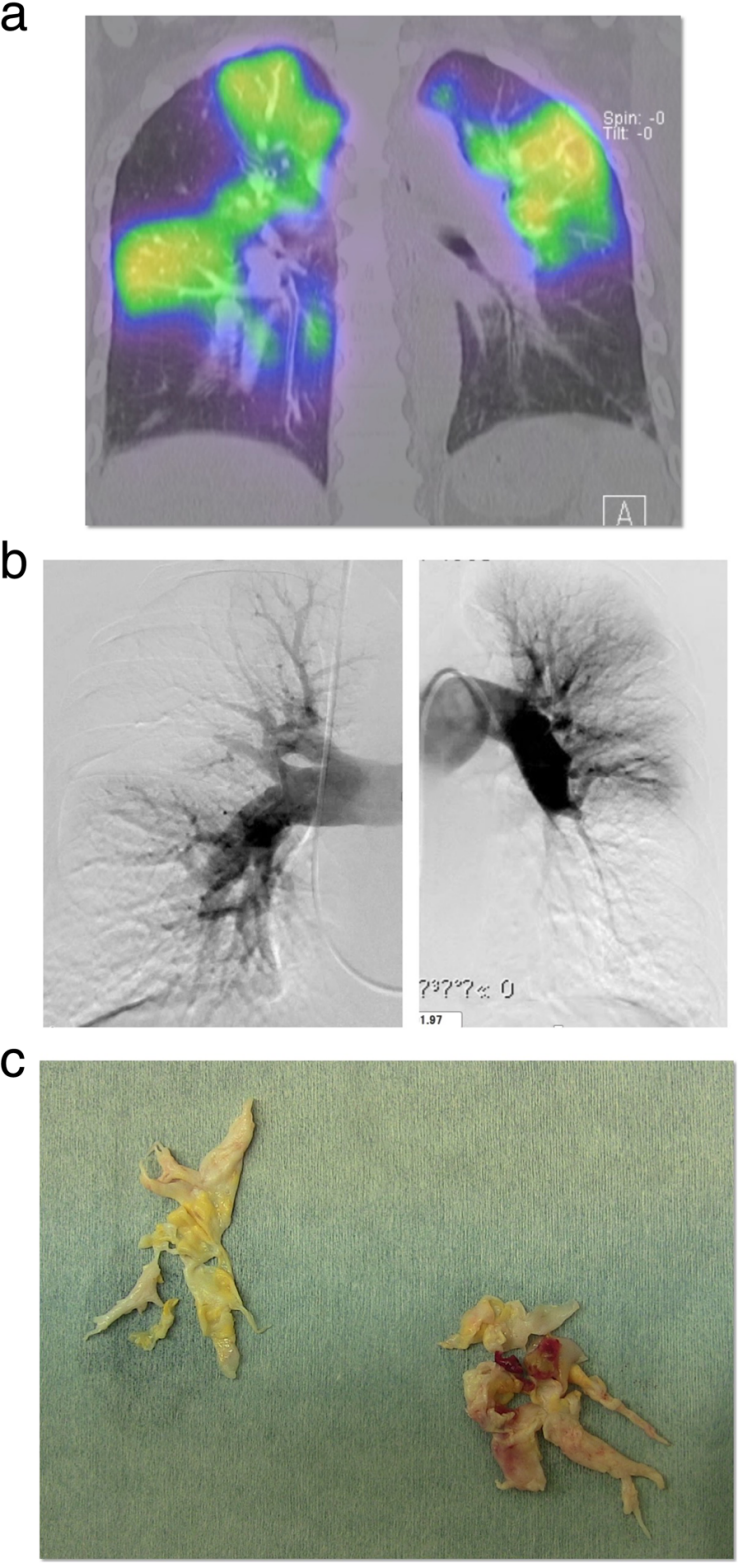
a. Preoperative lung perfusion scintigraphy showing multiple segmental defects. b. Preoperative pulmonary artery angiograms showing intimal irregularities and abrupt narrowing of both pulmonary arteries. c. Resected thromboembolism of both pulmonary arteries
